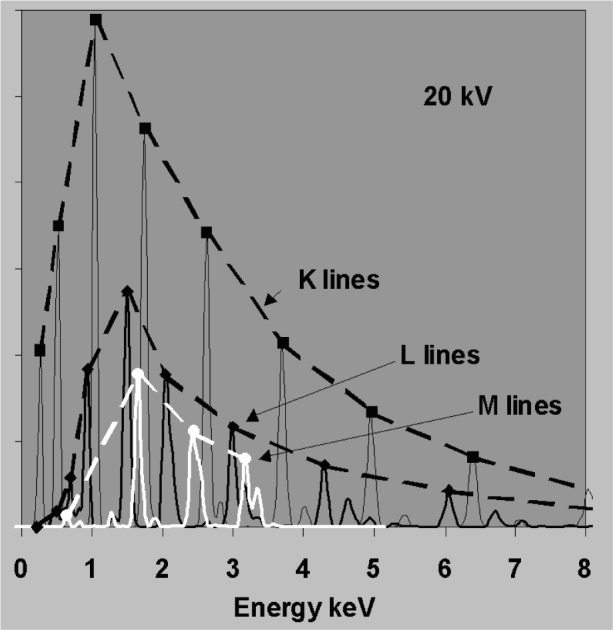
Relative intensities of x-ray lines excited in bulk pure elements at 20 kV for a Si(Li) detector with resolution 133 eV fwhm at 5.9 keV. The maximum peak intensity for K, L, and M series lines is shown as a function of x-ray energy to demonstrate that for the same concentration level, M and L lines are less intense than K lines.
